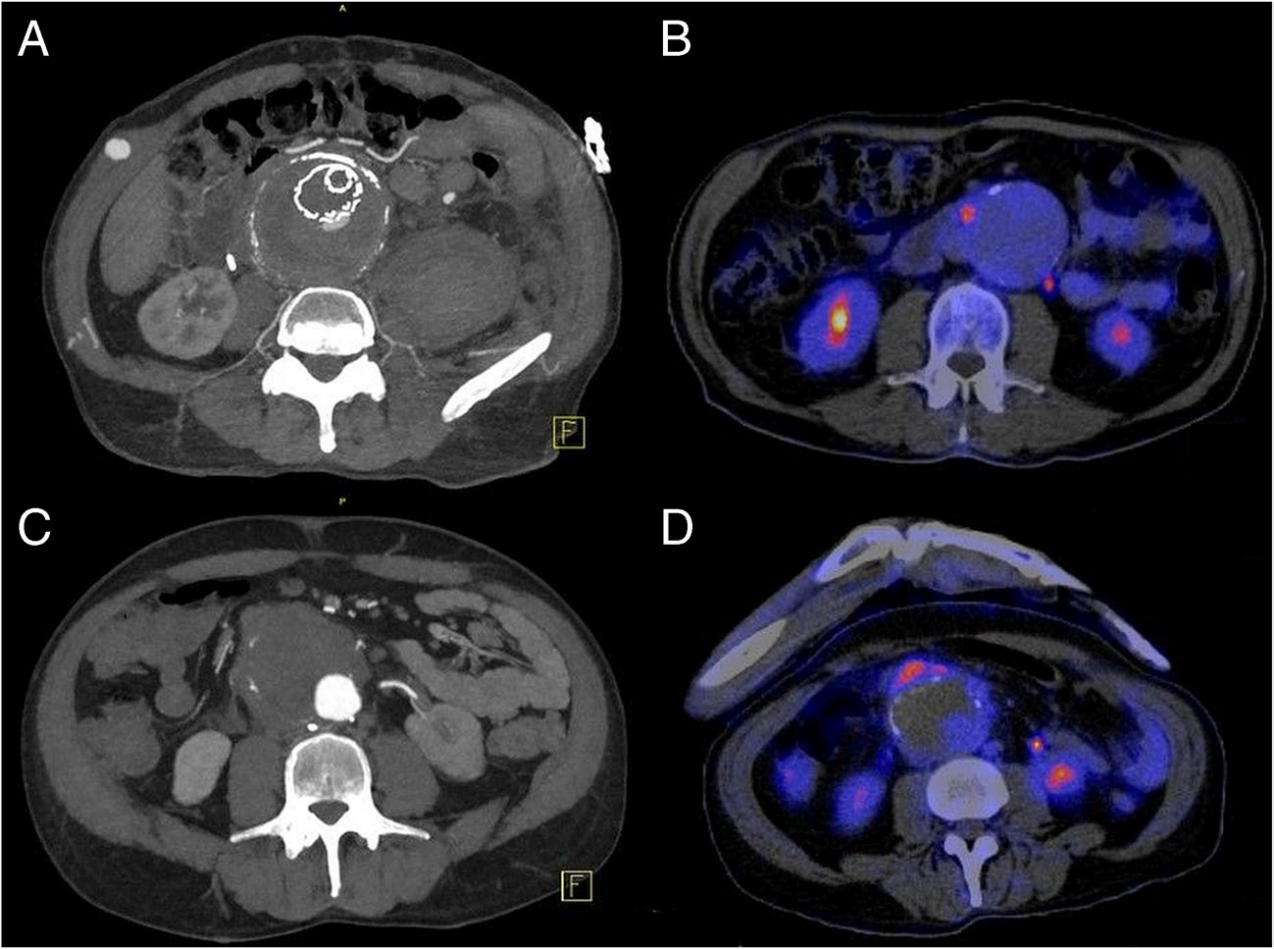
Imaging findings in Q fever aortitis. Panel A: Computed tomography angiography (CTA) demonstrating a ruptured aortic aneurysm with vascular endograft in case 2. Panel B: Fluorodeoxyglucose avid (FDG) avidity on positron emission tomography (PET) of the aneurysm wall in case 3. Panel C: CTA demonstrating 84 mm infrarenal aortic aneurysm with radiological signs of anterior aortic wall inflammation in case 4, with demonstrated FDG avidity in the aneurysm wall on the corresponding PET (panel D)
